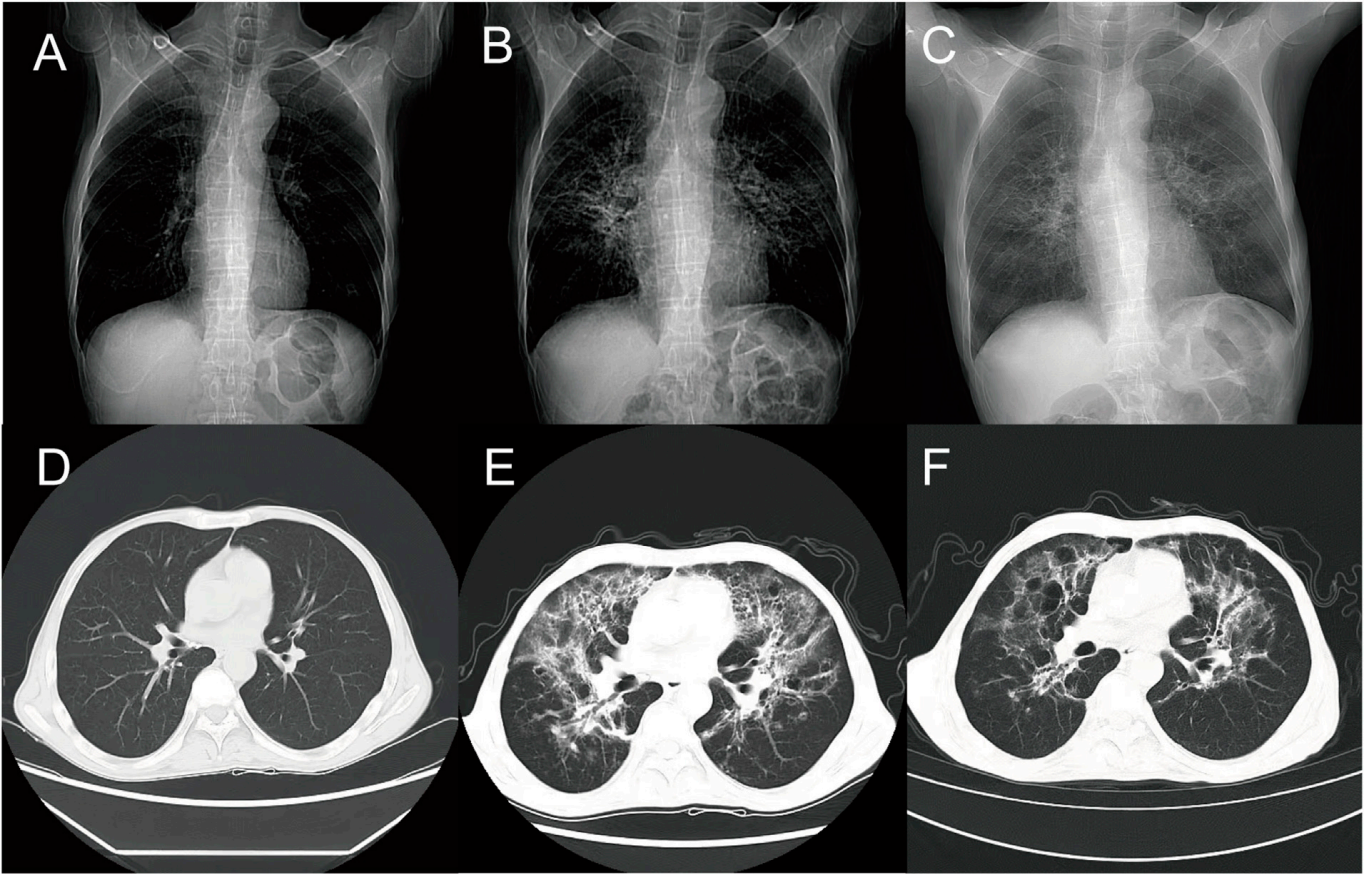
Chest CT. (A, D) After nine courses of combined treatment with oxaliplatin and capecitabine, the lung markings became clear, with minor visible streak-like and faint patchy high-density opacities. (B, E) Following one course of combined treatment with sintilimab and tegafur, the lung density decreased, revealing multiple streak-like, ground-glass, and patchy high-density opacities with ill-defined borders and uneven density, presenting a grid-like and cast-like change. (C, F) After discontinuation of antitumor drugs and administration of hormonal therapy, the lung density further decreased, showing multiple streak-like, ground-glass, and patchy high-density opacities with ill-defined borders and uneven density, presenting a grid-like and cast-like change.
